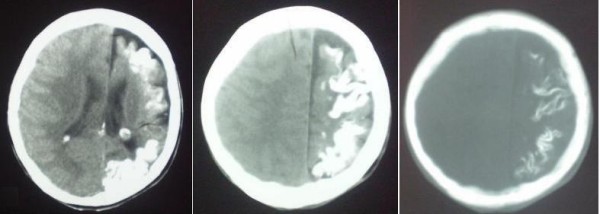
Cranial Computed Tomography shows cortical and sub cortical gyriform calcifications of the left brain hemisphere and cortical atrophy.
